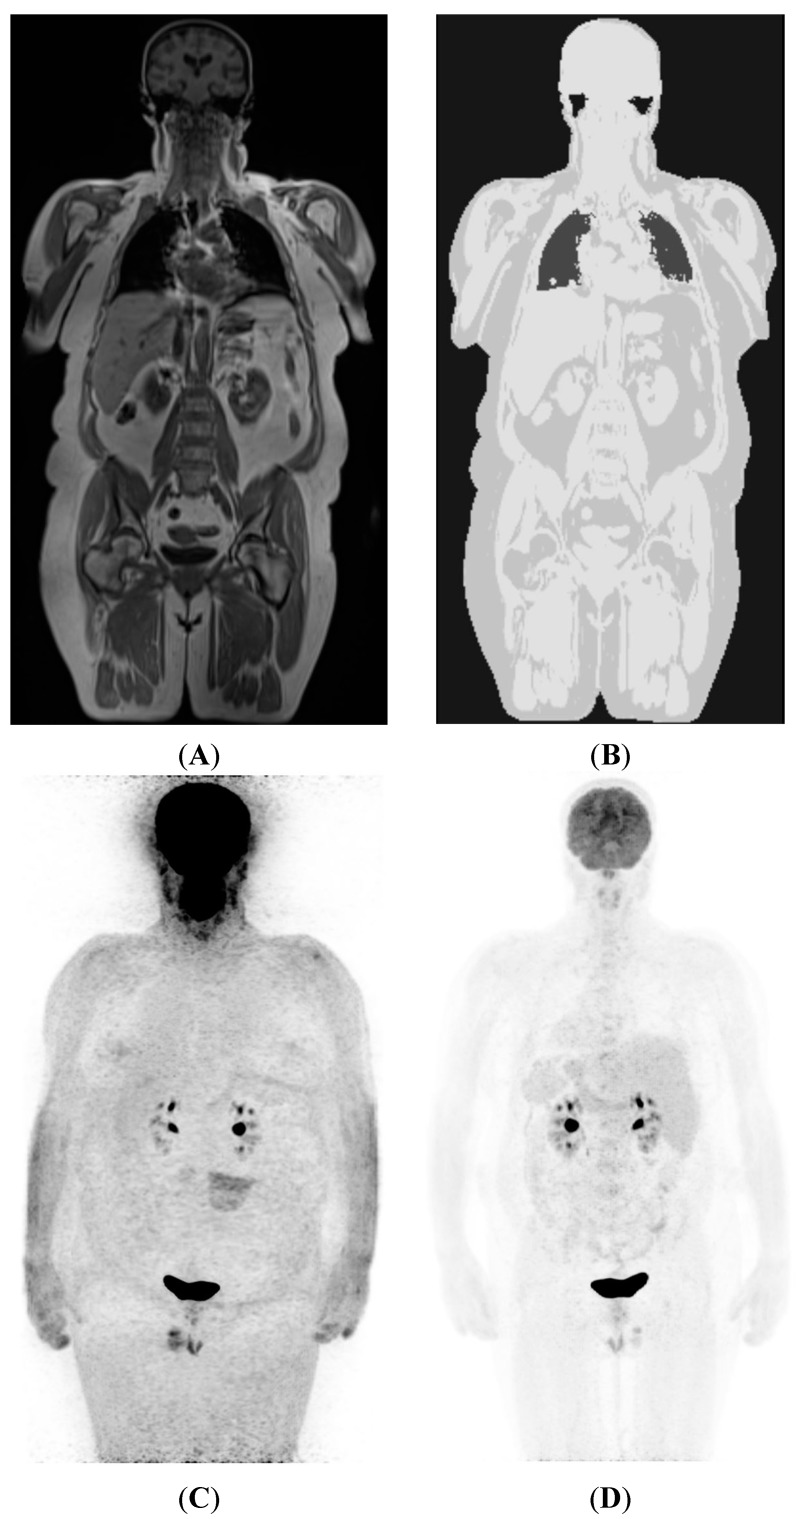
(A) Coronal plane image from the Dixon VIBE sequence used to generate a MR AC map; (B) shows attenuation map generated from (A). Note four gray levels separating air, lung, fat, and soft-tissue. Also note that while bone marrow appears similar to fat elsewhere, bone cortex and overlying muscles have been assigned similar attenuation values. Reliable separation of bone in AC maps is an area of active research; (C) A non-corrected ^18^F-FDG PET image, showing non-uniform tracer distribution with spuriously higher uptake in peripheral tissues due to greater attenuation of emission photons from deeper structure; (D) AC-corrected image after applying MRI-based attenuation map shown in (B).
